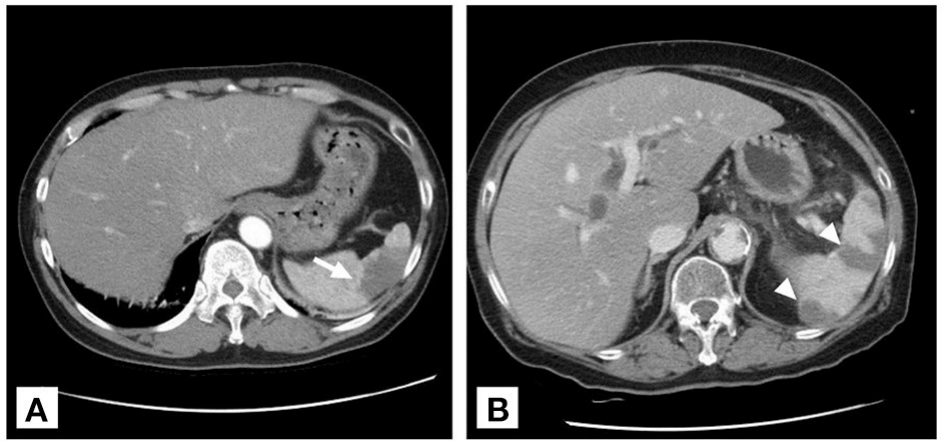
Two patterns of splenic infarction on contrast-enhanced CT. (A) Wedge infarct (arrow) in a 49-year-old male with celiac trunk dissection. (B) Multiple infarcts (arrowhead) in an 80-year-old woman with acute pancreatitis.
